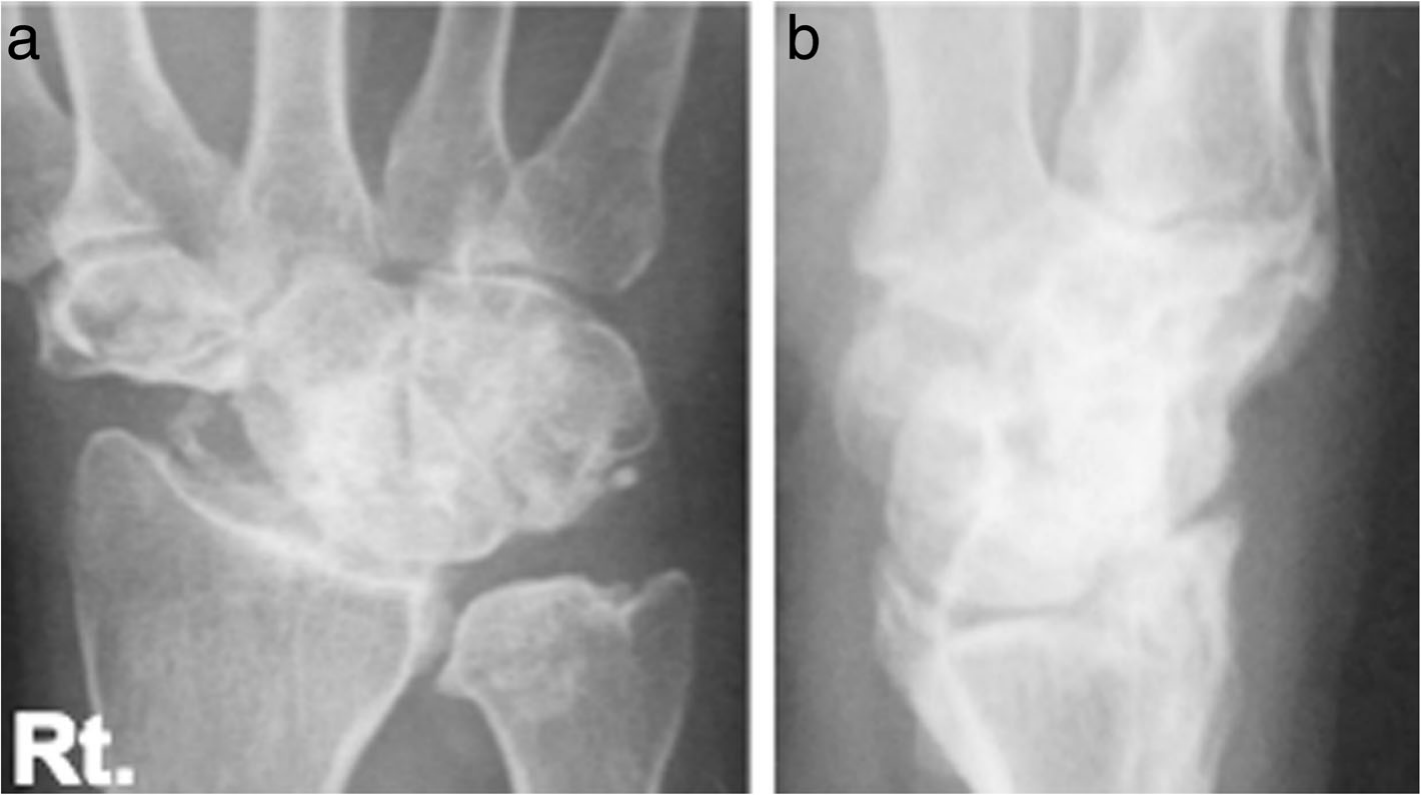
X-ray showing fully united (a, b) four corners
